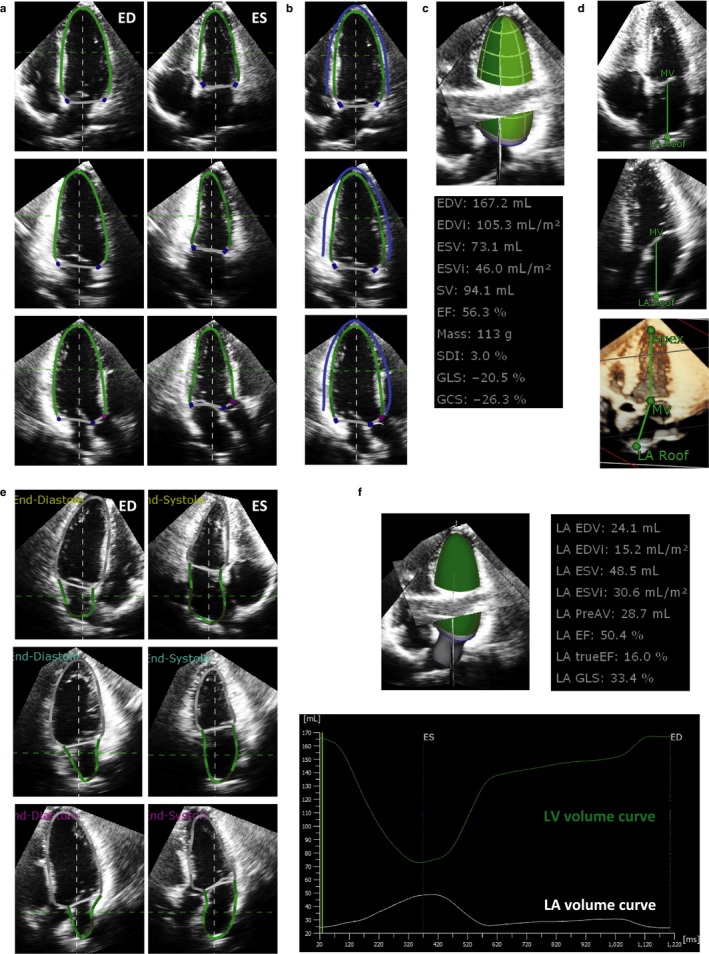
Step‐by‐step approach of left ventricular and left atrial volume measurements. (a) Left ventricular (LV) endocardial border tracing (green lines) on apical four‐chamber (upper panels), two‐chamber (middle panels), and long‐axis (lower panels) views at end‐diastole (ED) and end‐systole (ES). (b) LV mass calculation. Blue contour represents LV epicardial casts, from which LV mass was determined as (LV epicardial cast volume − LV endocardial cast volume) × 1.05. (c) Achievement of LV geometrical and functional assessment. (d) Initialization of left atrial (LA) endocardial contour. The center of the mitral valve and LA roof was determined on apical four‐chamber and long‐axis views. Note that the LV long axis and LA long axis lines are not parallel but angulated. (e) LA border determination on apical four‐chamber (upper panels), two‐chamber (middle panels), and long‐axis (lower panels) views at end‐diastole (ED) and end‐systole (ES). (f) Results of LA geometrical and functional assessment. LV and LA volume curves during one cardiac cycle are also shown
